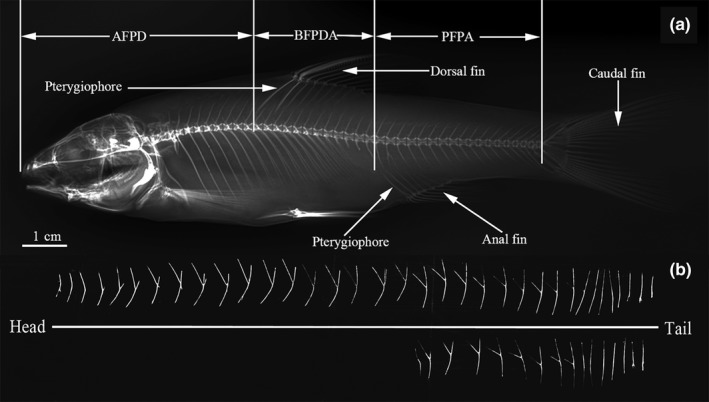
The intermuscular bone (IB) counts from X‐ray photography and anatomy of *Sinocyclocheilus grahami*. (a) lateral view of radiograph with labels dividing regions of a fish; (b) lateral view of IBs that were extracted from a dissected fish
